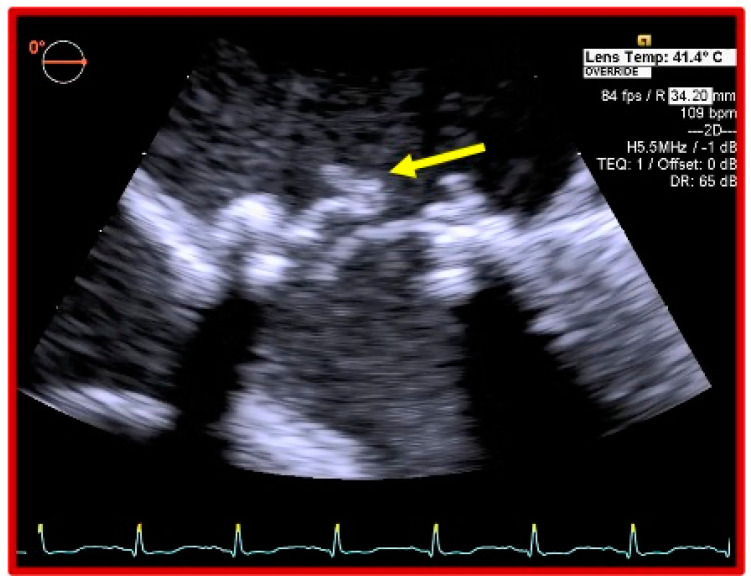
Two-dimensional transesophageal ECHO showing a flail leaflet of the bioprosthetic mitral valve.
